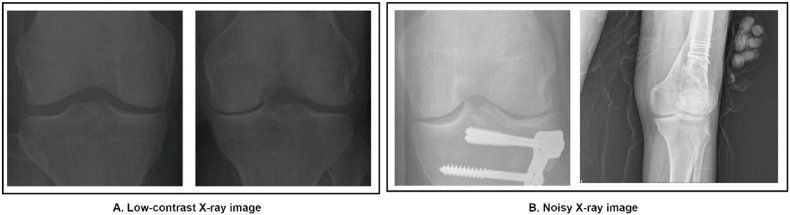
Challenges for the Knee X-ray images in the dataset: A. Low-contrast X-ray image and B. Noisy X-ray image.
